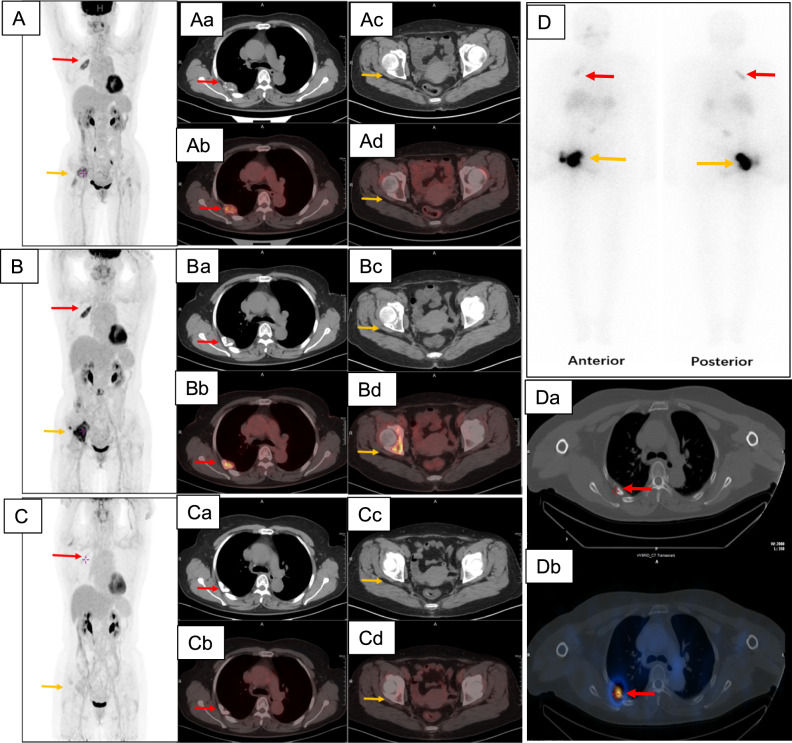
In case 5, ^18^F-FDG PET/CT showed bone (right 5^th^ rib and right acetabulum) metastases before apatinib therapy (A and Aa to Ad). Increased tumour sizes and a new metastasis formation in the right ilium were revealed after 6-month therapy with apatinib (B, Ba to Bd). After combined therapy with RAI, decreases in tumour sizes and SUVmax were shown (C, Ca to Cd). The post-therapeutic ^131^I whole body scans in anterior and posterior views (D) and CT (Da) as well as SPECT/CT (Db) of 5^th^ rib metastases were shown. Red arrows indicated metastasis in the right 5^th^ rib and orange arrows metastases in the right acetabulum
